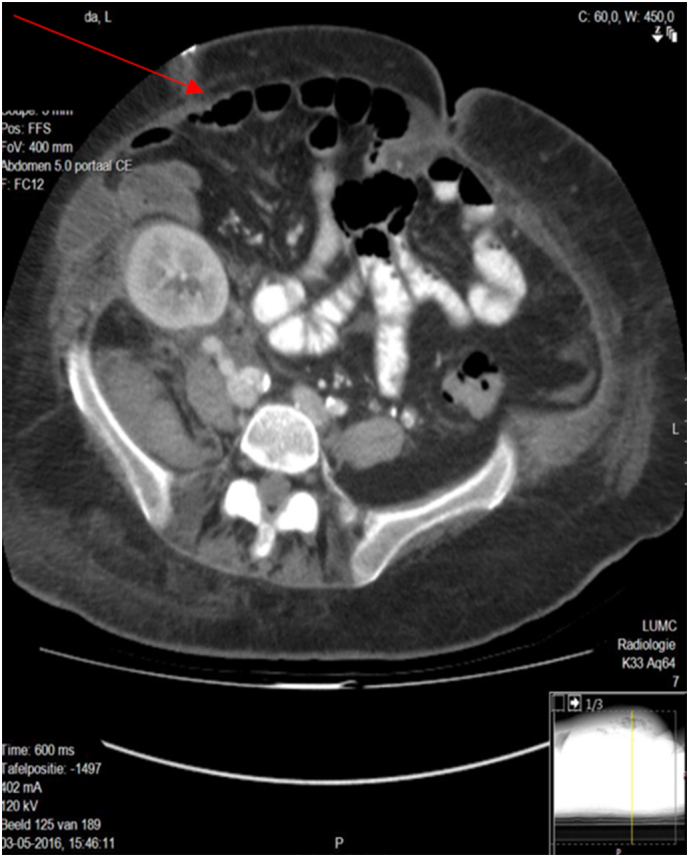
A transverse section of an abdominal Computed tomography (CT) showing the LIH (red arrow).
